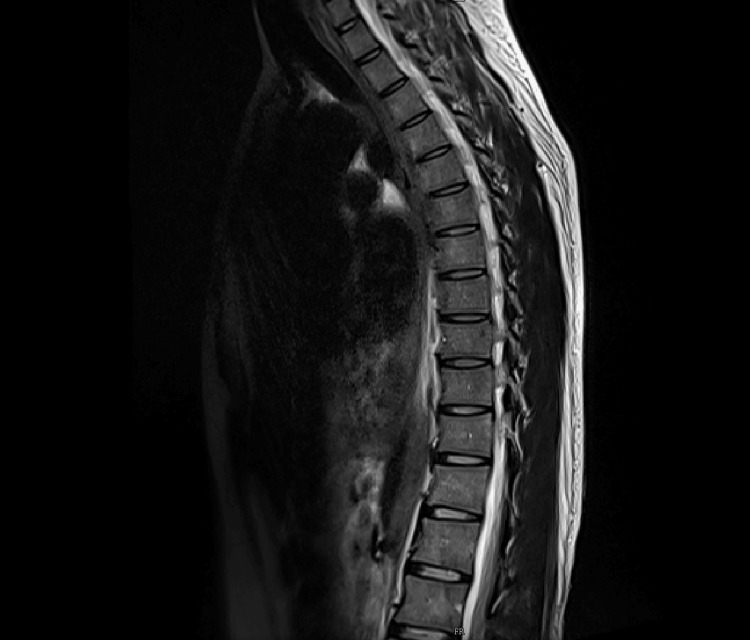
Sagittal MRI sequences of the thoracolumbar spine demonstrating no significant abnormalities.
